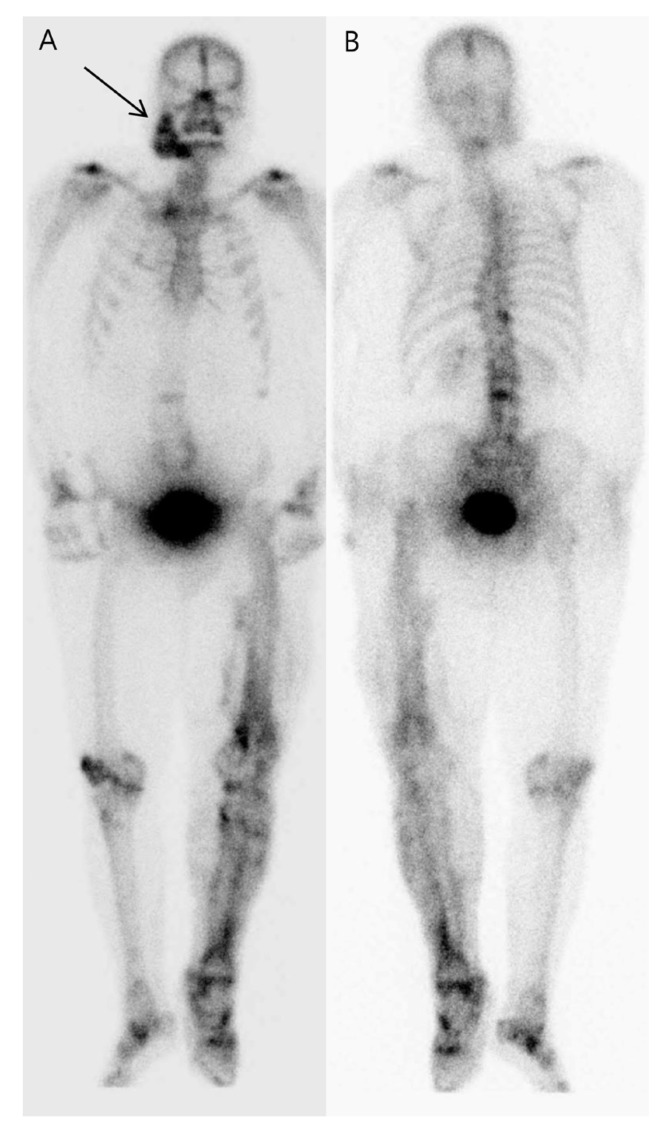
The whole-body bone scan was obtained 4 h after the intravenous injection of 740 MBq of technetium-99m methylene diphosphonate (MDP), using a gamma camera with a gantry motion of 10 cm/min and a matrix size of 256 × 1024. It showed marked increased radiotracer uptake in the central photopenic area in the right mandible. Multiple abnormal soft tissue radioactive uptake lesions were observed at the level from the left mid-to-distal thigh to the left foot, and the radioactivity uptake was slightly increased in the lower thoracic spine and the L3 spine. Based on these findings, fibrous dysplasia or an odontogenic tumor was first considered for the right mandible lesion. The possibility of heterotopic ossifications was considered for the uptake lesions in the soft tissue of the left lower extremity, which were consistent with the distribution of abnormal calcified opacities seen in the conventional plain radiograph. In the case of spinal lesions, when referring to X-ray findings, it was judged to be caused by degenerative disc disease and compression fracture due to scoliosis, although it was early onset compared to the patient’s age. Both anterior (A) and posterior (B) images of the whole-body bone scan revealed significantly increased MDP uptake in the left lower extremity. There was a hyperactive bone lesion with a central photopenic defect in the right mandible (arrow). In addition, there were increased radio-uptakes suspected to be degenerative lesions caused by scoliosis in the spine.
